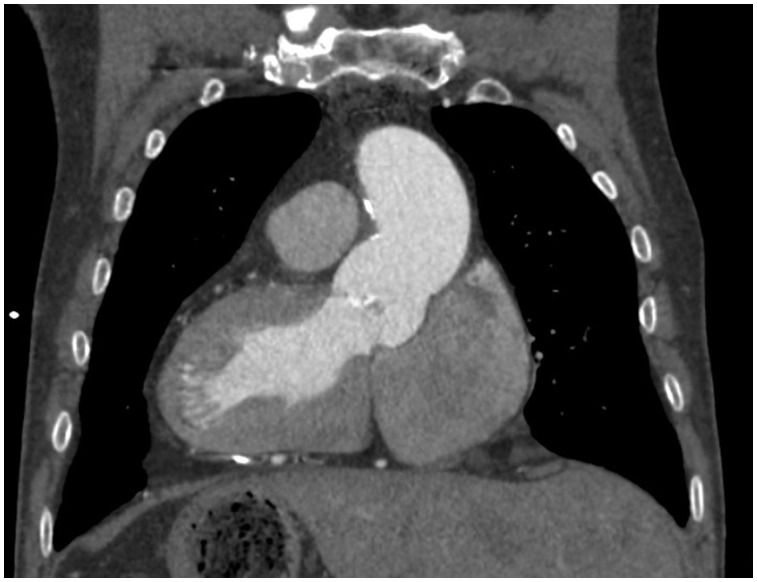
Transversal view of a computed tomography scan of the complete situs inversus.
